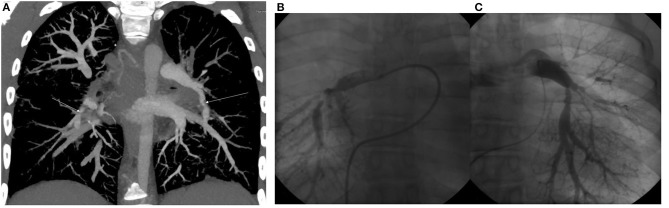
(A) CT angiography. Maximum intensity projection in coronal view. Arrows show severe segmental stenoses in the lower right and left lobes. (B) Selective pulmoangiography of the right pulmonary artery. We can see severe segmental stenoses of all right lower lobe segments. (C) Selective pulmoangiography of left pulmonary stenoses. All segments with moderate to severe stenoses. Decreased arborization pattern of left pulmonary vascular bed.
